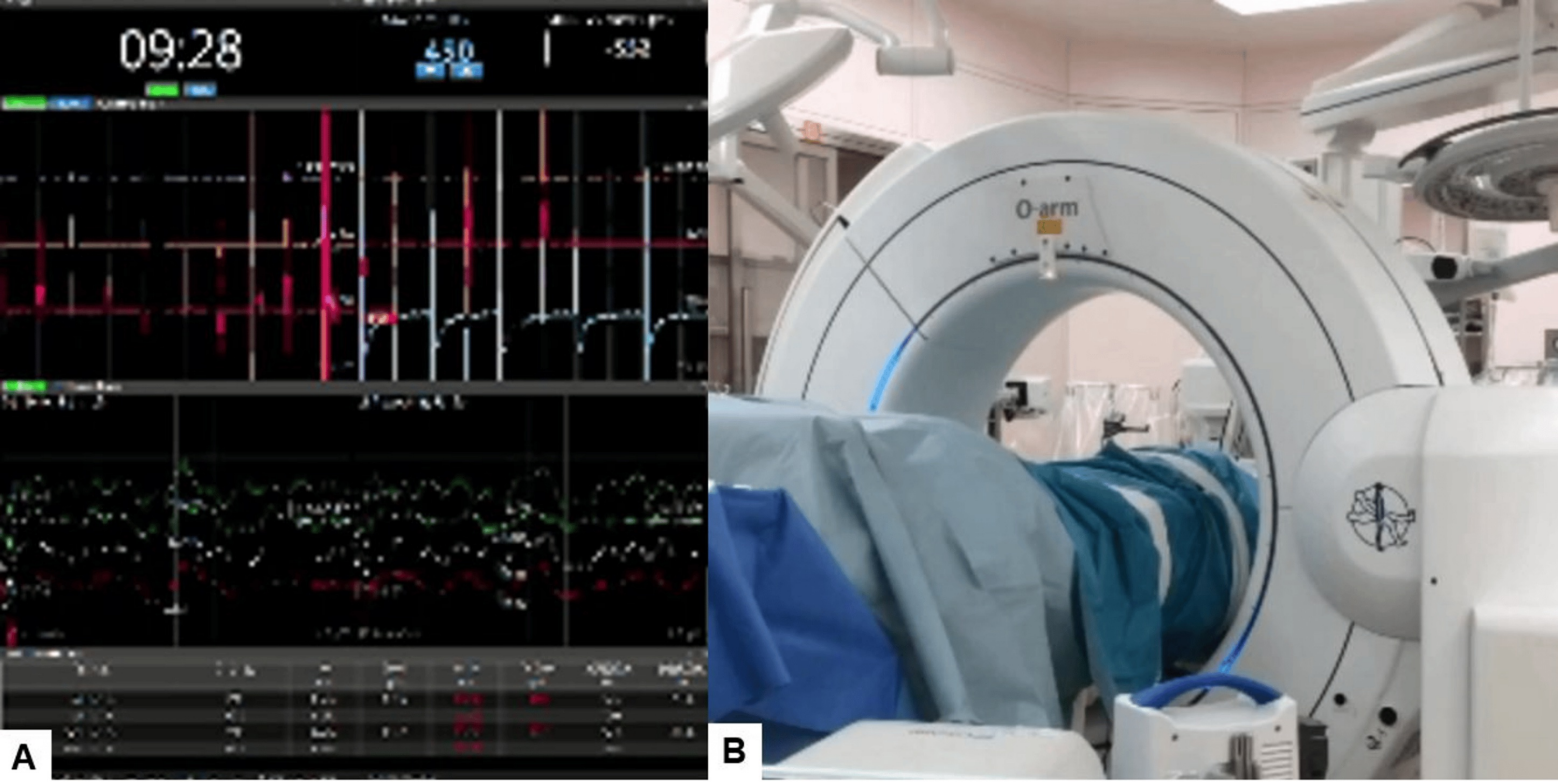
Neuromonitoring and O-arm (A) Continuous neuromonitoring was initiated at the beginning of the procedure. (B) A CT scan was obtained using the O-arm to provide detailed imaging guidance.
